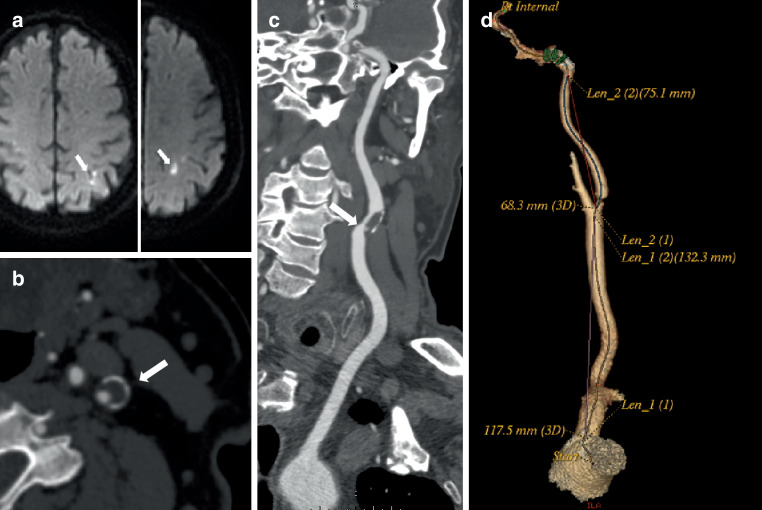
Carotid computed tomography angiography (CTA) imaging markers and stroke work-up. This 73-year-old man presented with abrupt onset right-sided weakness and numbness. Axial diffusion-weighted imaging (a) showed an acute infarct (*long arrows*). Axial CTA of the carotid arteries (b) demonstrated a thick left proximal internal carotid artery plaque with a positive rim sign (*long arrow*). Multiplanar reformat of CTA images (c) demonstrated the ulcerated carotid plaque (*long arrow*). Three-dimensional volume rendering (d) showed the linear distance of the vessel was determined as the shortest distance between the same two points, the actual distance of the vessel was measured from the proximal to distal point along the arterial center line from the curved projection reformation
